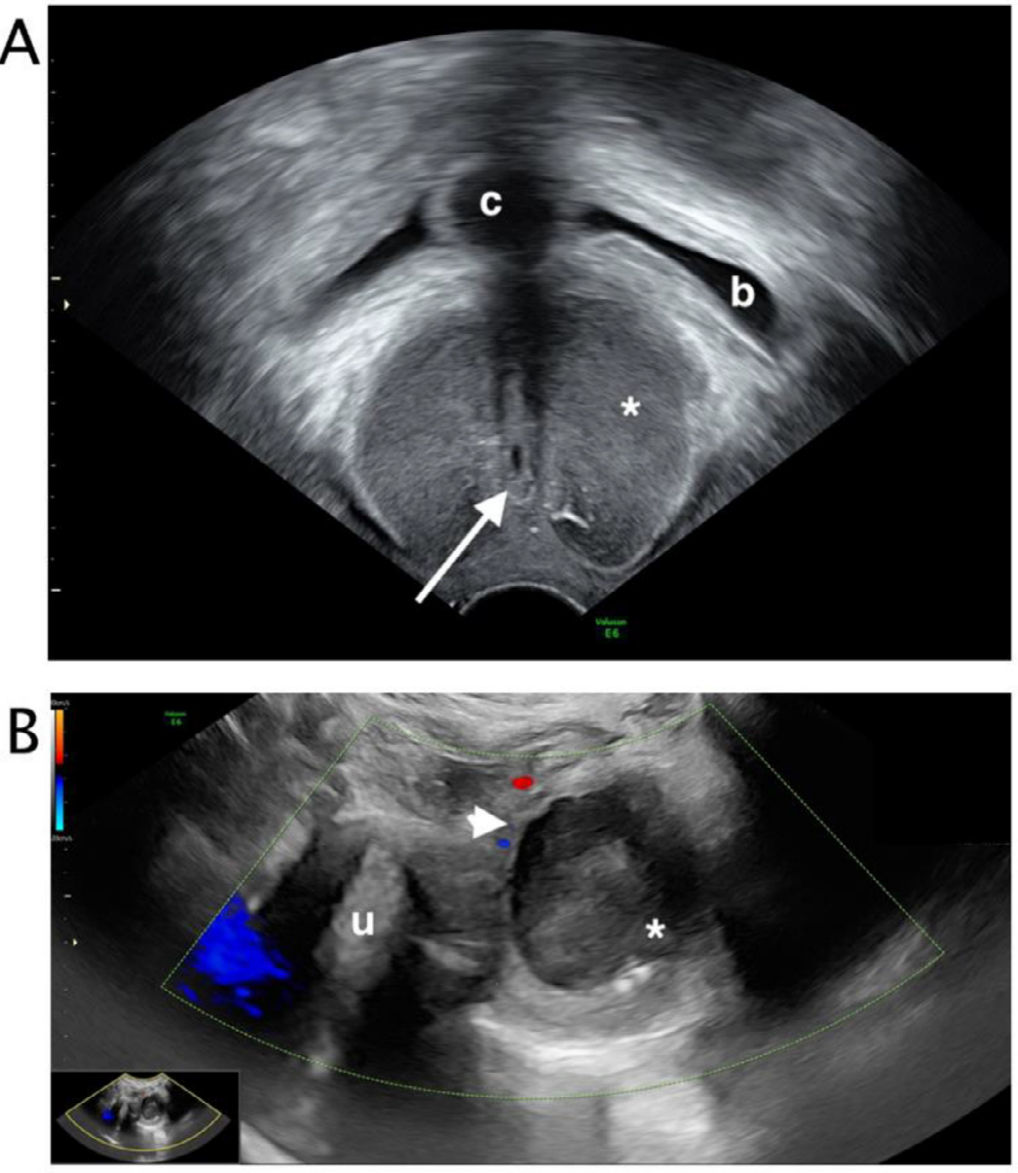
Transvaginal ultrasound. At transvaginal ultrasound, a bilocular cyst with a non-vascularized septum (white arrowhead) (B), low level content and regular walls (white asterix), was visualized below the catheterized (c) bladder (b) and anterior lateral to the urethra (white arrow) (A). There was no communication between the cyst and urethra. Uterus (u).
